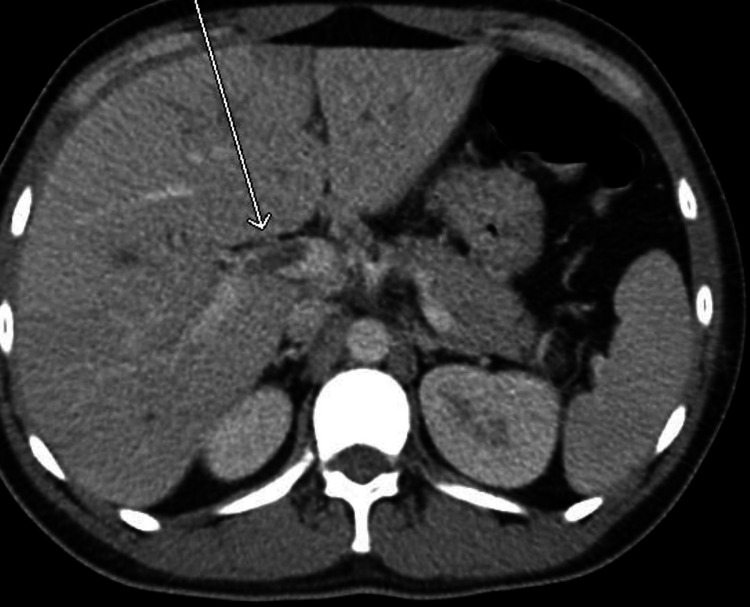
CT abdomen (axial view) showing portal vein thrombosis
